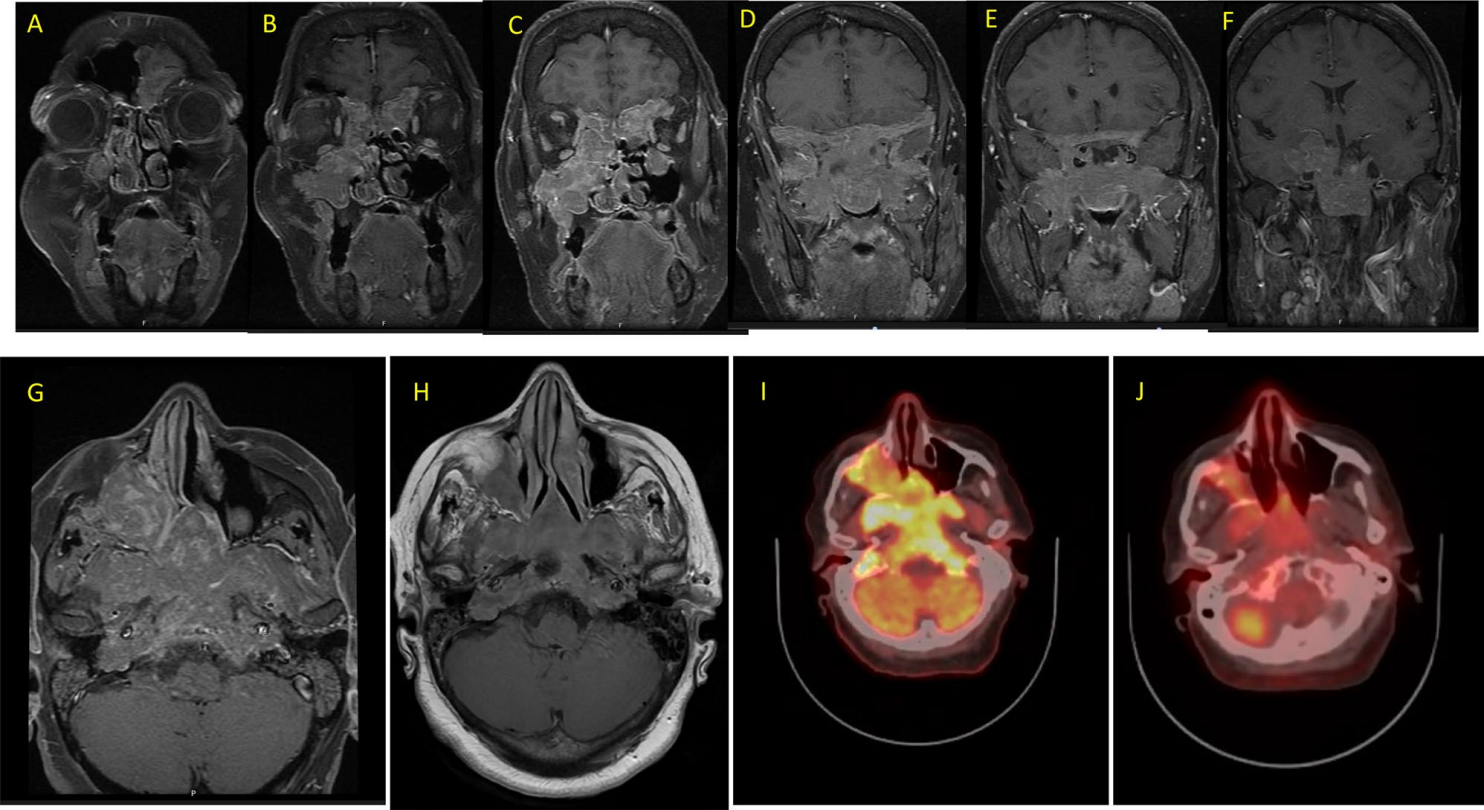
The upper panel (A–F) are serial coronal sections of a gadolinium-enhanced T1 images showing a huge enhancing mass involving the sellar, parasellar and suprasellar region and extending inferiorly to the sphenoid and paranasal sinuses, nasopharynx and nasal cavity, especially on the right side. It extends laterally and posteriorly to the middle and posterior fossa. In the lower panel, these axial sections show the mass before starting therapy (G) and after 7 months on cabergoline therapy (H) showing a major reduction in the size and enhancement. The same is also demonstrated on FDG PET CT scans done before (I) and 7 months after starting cabergoline therapy (J) revealing a major reduction in the size and avidity of the giant prolactinoma that this patient had
